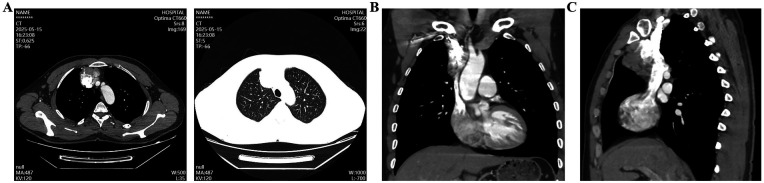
Enhanced chest CT revealed a mediastinal space-occupying lesion. (A) Mediastinal window and lung window; (B,C) Coronal and sagittal positions.
